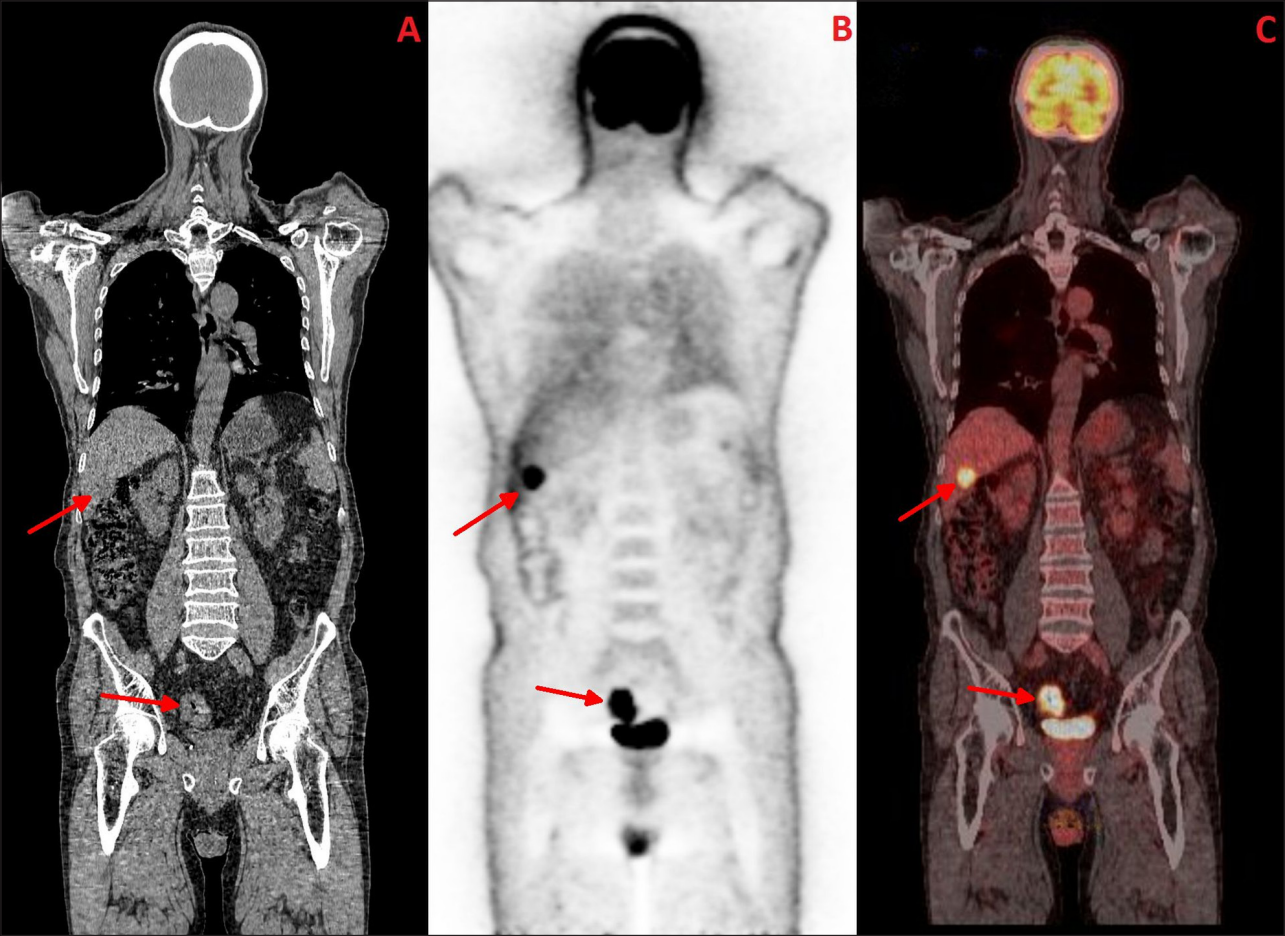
Coronal plane; A- CT image revealing the rectal and liver lesions; B- PET image; C- Fused PET-CT image.
